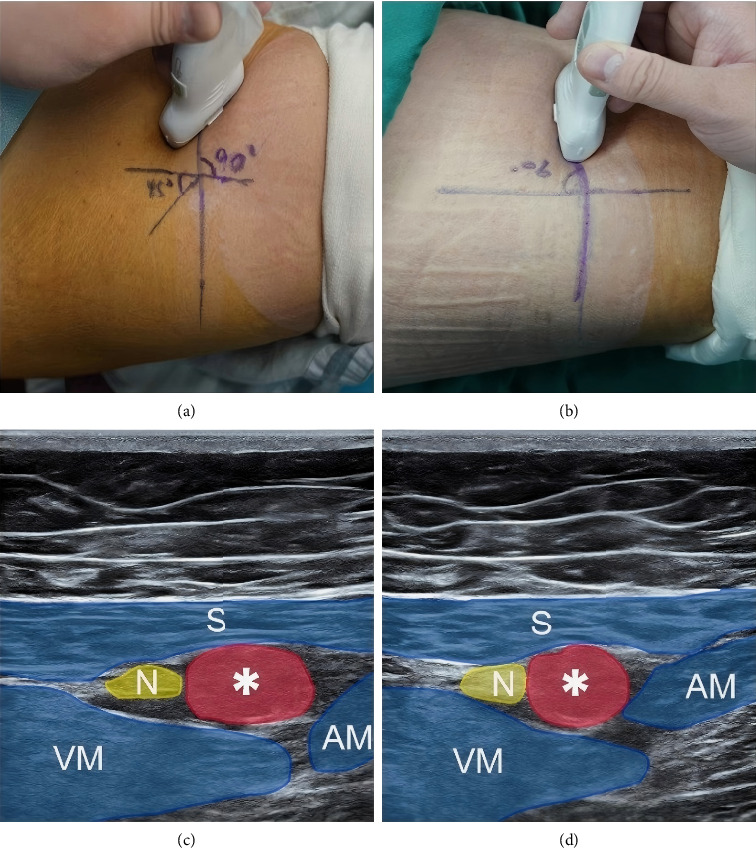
Legends: the oblique approach was inserted at the position of (a), and the brachyaxis approach inserted at the position of (b). Ultrasound images of the (c) oblique approach of adductor canal and (d) the brachyaxis approach of adductor canal. ^∗^, femoral artery; N, saphenous nerve; S, sartorius; VM, vastus Medialis; AM, adductor magnus.
